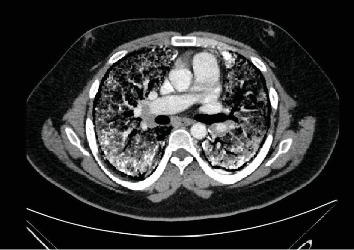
Chest CT scan with diffuse ground glass opacities and punctate calcifications involving bilateral lungs.
